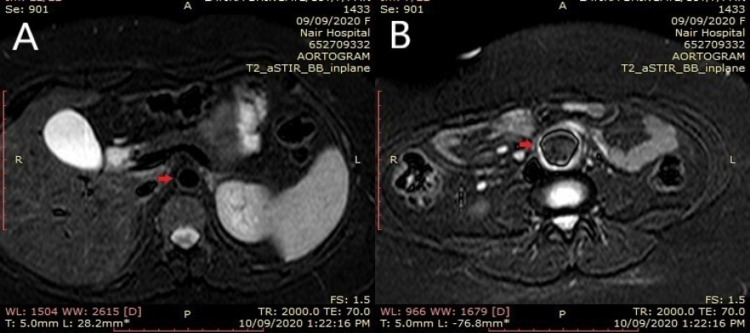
MRI aortogram MRI images showing wall thickness (red arrow) and enhancement in the suprarenal part (B) as compared to the normal aorta (red arrow) (A)
